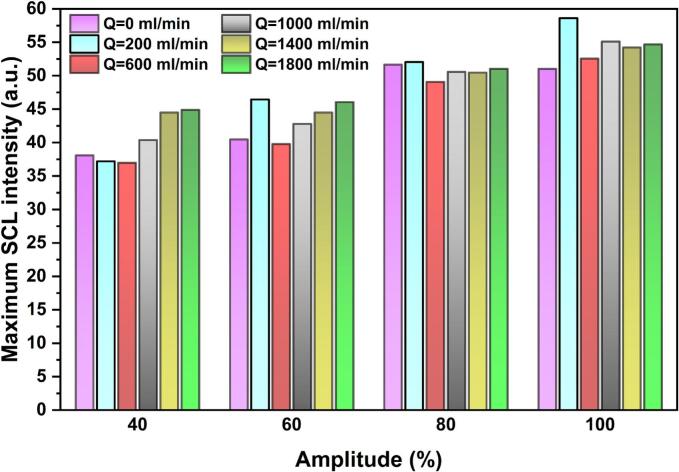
Maximum SCL intensity under various flow rates and ultrasonic amplitudes in co-current configuration.
